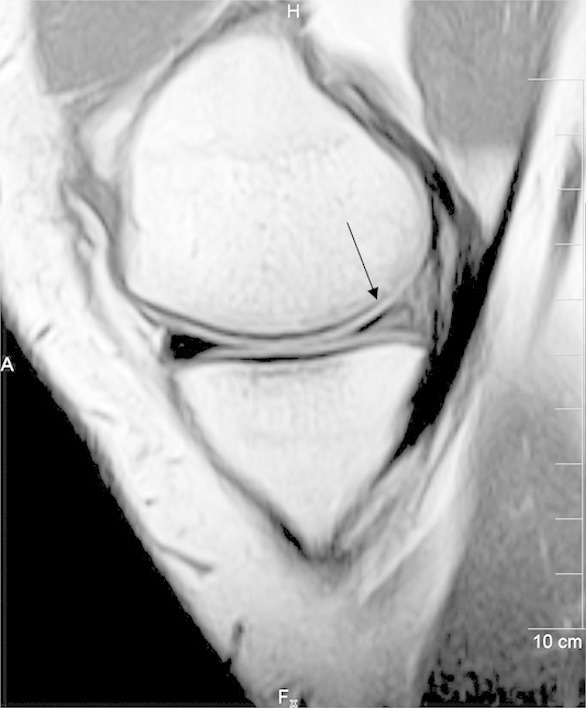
Sagittal T1-weighted MR image showing Grade-3a tear in the posterior horn of medial meniscus that extends to the inferior articular surface. (Black arrow) On arthroscopy the tear was not documented performed 14 days after MR imaging.
